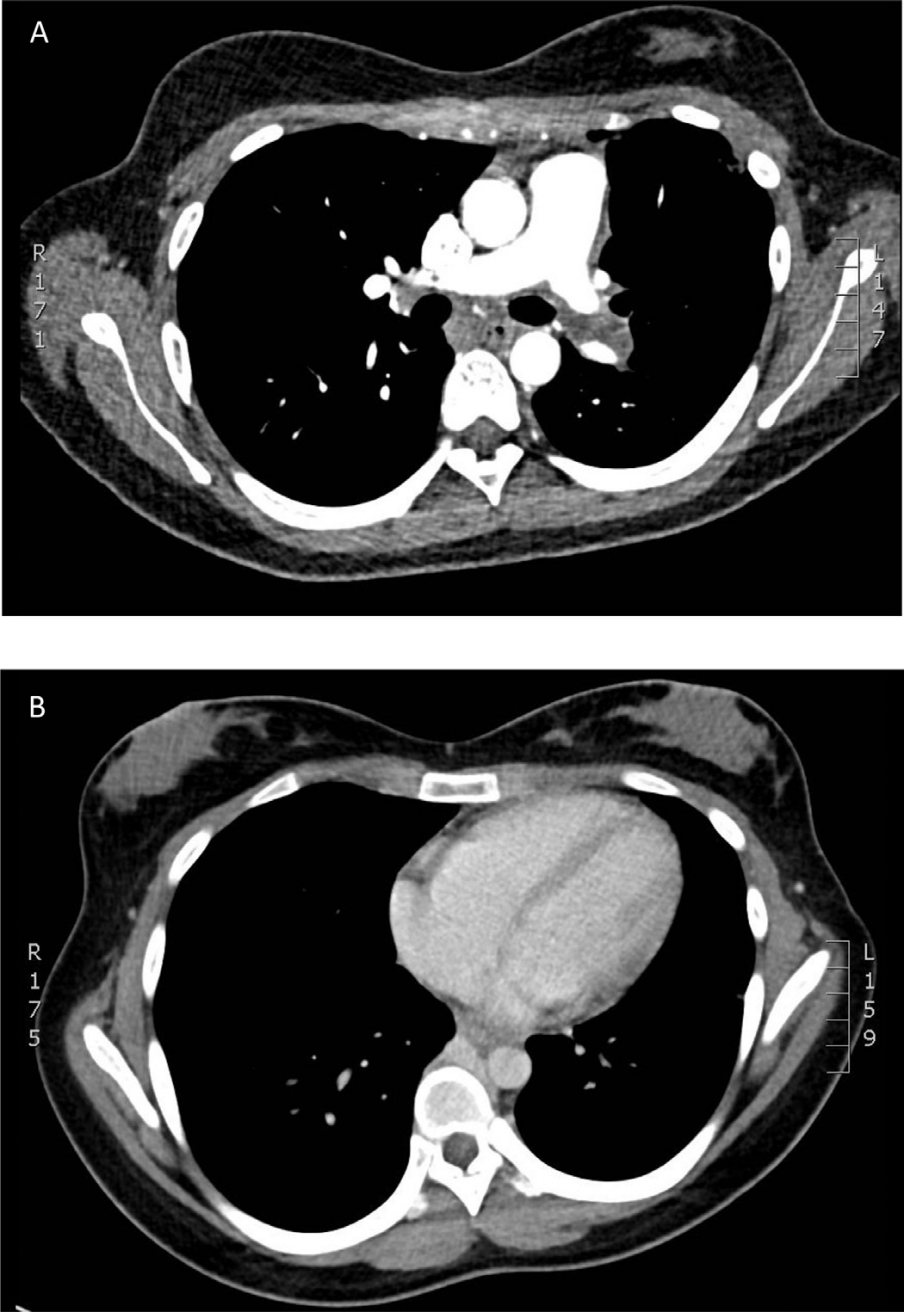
Follow-up computed tomography pulmonary angiogram (CTPA) showing (a) some improvement to the left pulmonary arterial flow and (b) right atrium free of thrombus and resolution of the radiographic findings of right heart strain.
